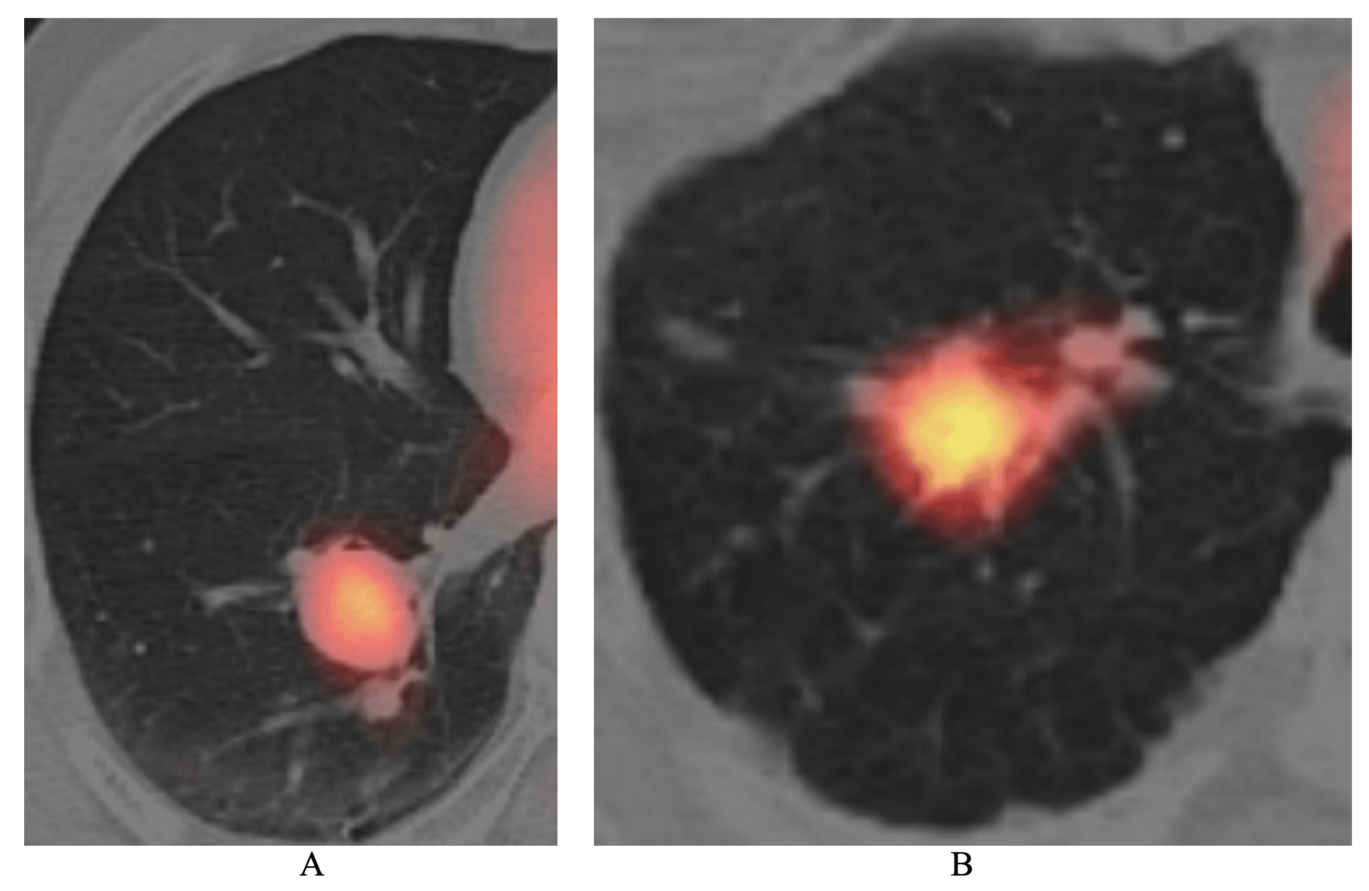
Positron emission tomography (PET) findings A. The maximal standardized uptake value (SUV max) of the tumor showed 3.76 g/mL just before operation. B. PET,  as a reference image, of the metastatic lung tumor from breast cancer showed a SUV max value of 10 g/mL despite the similar tumor size to this case.
